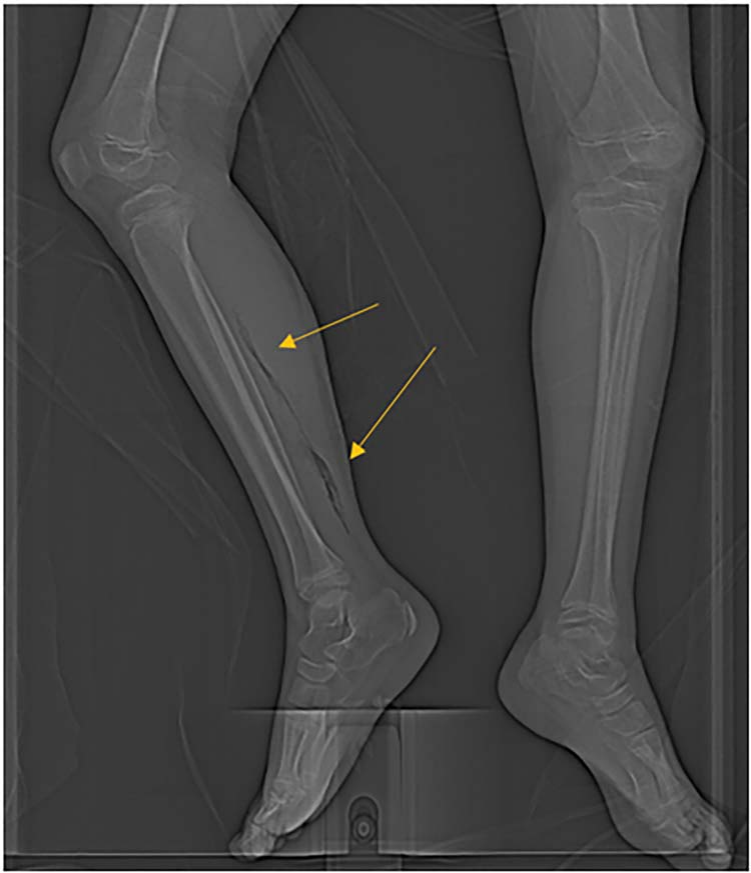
CT scan demonstrating extensive air in the soft tissue of the right leg.
